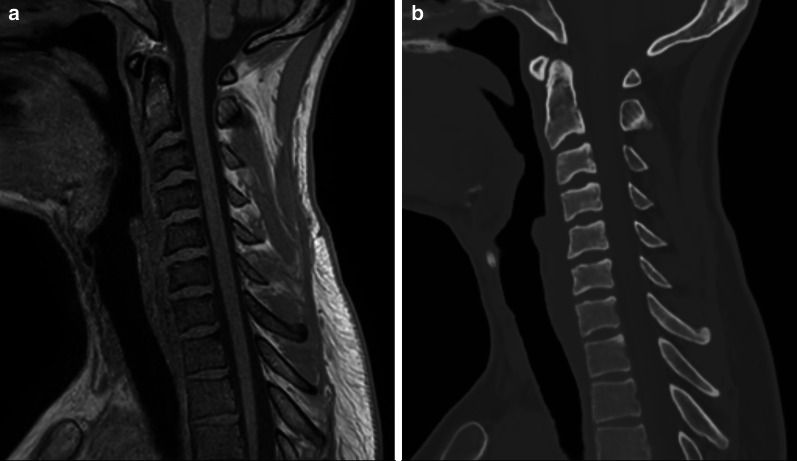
The CE-marked BoneMRI software product [16] (BoneMRI V1.1, MRIguidance BV, Utrecht, The Netherlands) has been provided as a self-contained application connected to the local PACS through the IMAGR platform. The figure shows T1-weighted MRI (left) and reconstructed BoneMRI (right) images of the cervical spine of a healthy volunteer. The 3D BoneMRI image is reconstructed from the T1wMRI images using the BoneMRI software and pushed back into PACS
